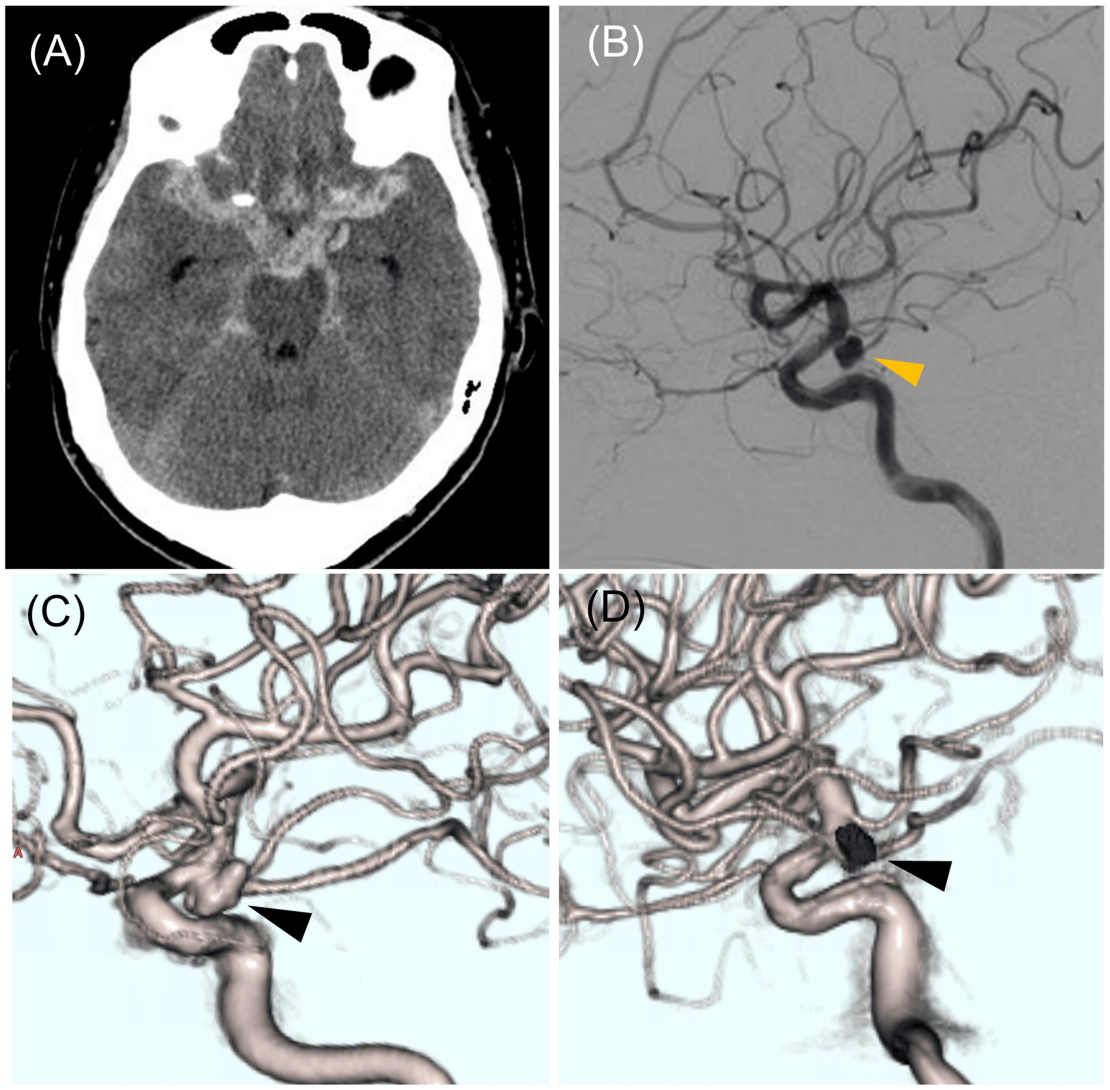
A case of subarachnoid hemorrhage due to the rupture of a left internal carotid artery aneurysm A left ICA aneurysm (AN) was found in a female in her 50s (Hunt and Hess grade IV) on head CT angiography, and endovascular treatment was performed. (A) CT image at the time of the visit. (B) Digital subtraction angiography image. The yellow arrowhead indicates AN. (C) Three-dimensional rotational angiography. The black arrowhead indicates AN; (D) Three-dimensional rotational angiography after coil embolization. The clump indicates coils (black arrowhead).
